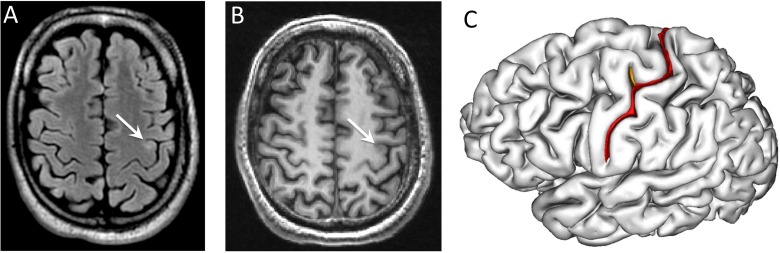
Visual identification of FCD sulci in a MR+ patient. The hyperintensities in FLAIR (A) and the slight cortical thickening visible in T1 MRI (B) allowed to locate the lesion in the depth of an ascending branch of the left central sulcus (white arrows), at the intersection of the main branch of the central sulcus. These two sulci are automatically labeled (C): « superior branch of the pre-central sulcus» (orange) and « central sulcus » (red) and considered as FCD sulci.
